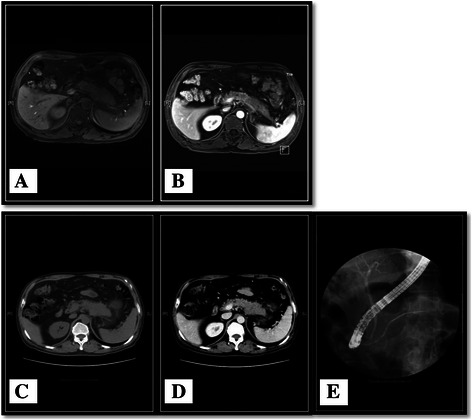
Imaging examinations. MRI showed enlargement of the pancreas body and tail with poor enhancement after gadolinium injection. Some enlarged peripancreatic lymph nodes were noted (A, B). Abdominal CT revealed diffuse enlargement of the pancreas body and tail, which appeared to be hypoattenuated with an unclear margin. A mass measuring 5.2 × 4.8 × 4.1 cm with probable encasement of the splenic vein was observed in the enlarged pancreas on contrast-enhanced CT (C, D). None of the imaging examinations revealed abnormal findings in the liver. The main pancreatic duct, common bile duct, and intrahepatic bile ducts were normal on endoscopic retrograde cholangiopancreatography (E).
